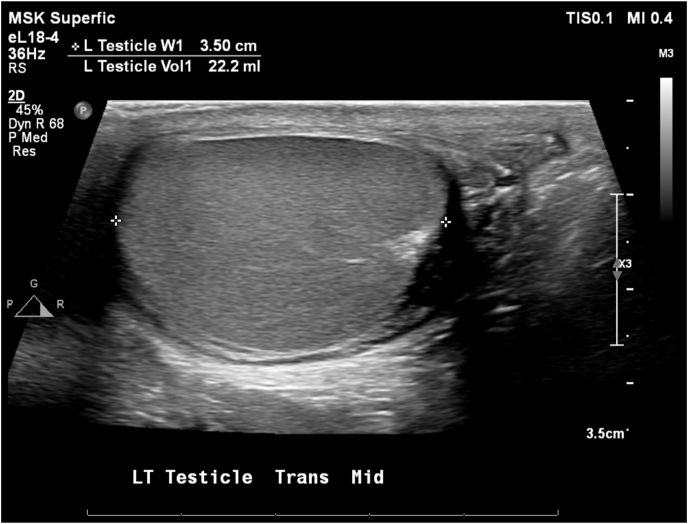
Ultrasound of left testicle
